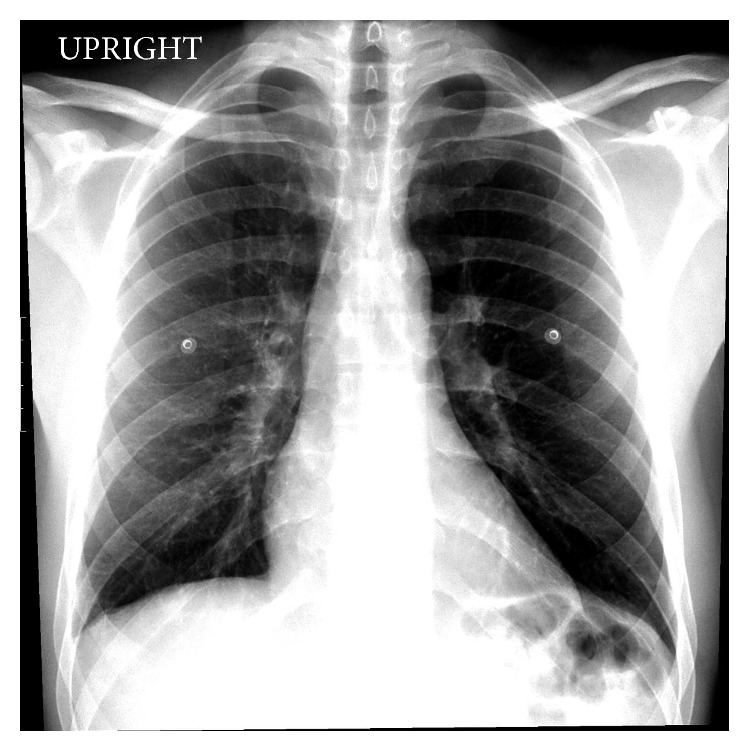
Chest X-ray showing no evidence of acute lung pathology.
